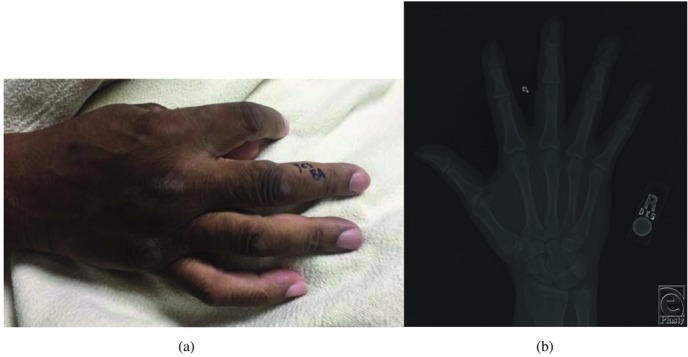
(a, b) Preoperative photograph and radiograph demonstrating a mass on the radial aspect of the right long finger. Radiograph demonstrates soft-tissue swelling, indicated by an arrow, without involvement of the proximal phalanx.
